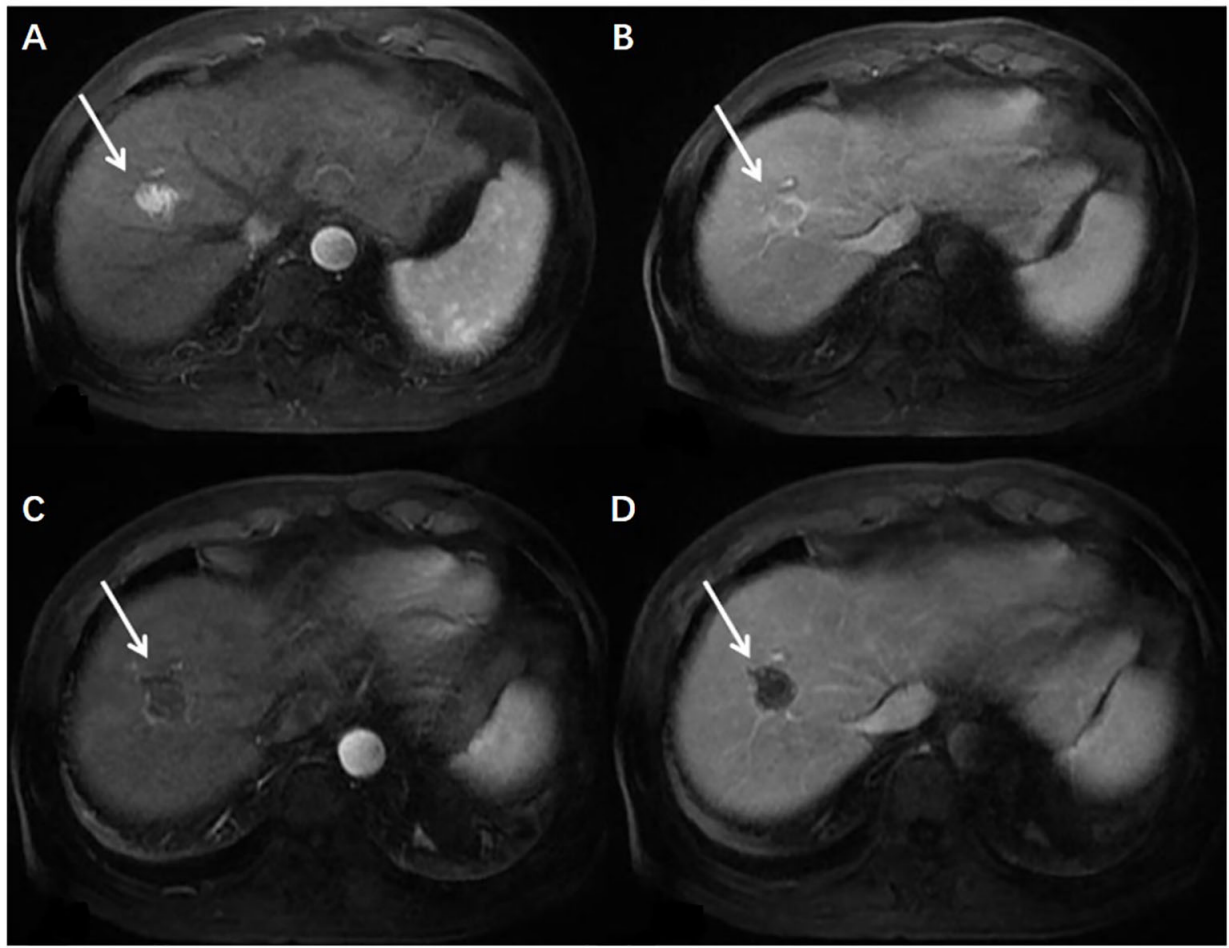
Changes in enhanced MRI images before and after HIFU in a 62-year-old male patient with HCC located in the right liver lobe (lesion size: 16 × 23 mm). (A) Before HIFU, the arterial phase in T1WI showed the rich blood supply in the lesion; (B) Before HIFU, the portal venous phase in T1WI showed partial contrast clearance in the lesion; (C) After HIFU, the arterial phase of T1WI did not show contrast perfusion in the lesion; (D) After HIFU, the portal venous phase in T1WI showed no contrast perfusion in the lesion.
